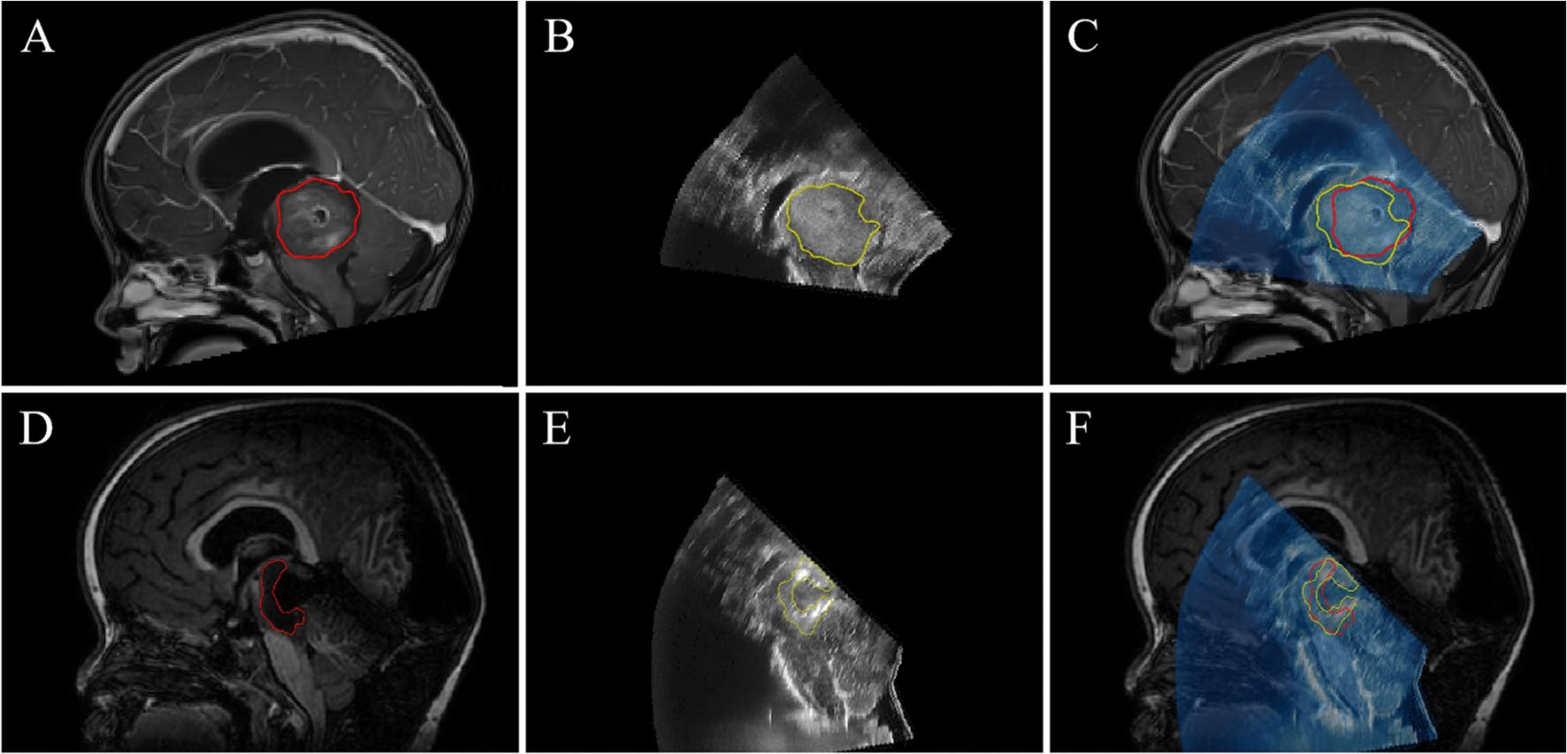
Example segmentations in preoperative and intraoperative MRI and US images. The first row shows the tumor segmentation in A preoperative MRI, B iUS1, and C for both modalities in an overlay. The second row shows the remnant segmentation in D intraoperative MRI, E iUS2, and F for both modalities in an overlay
